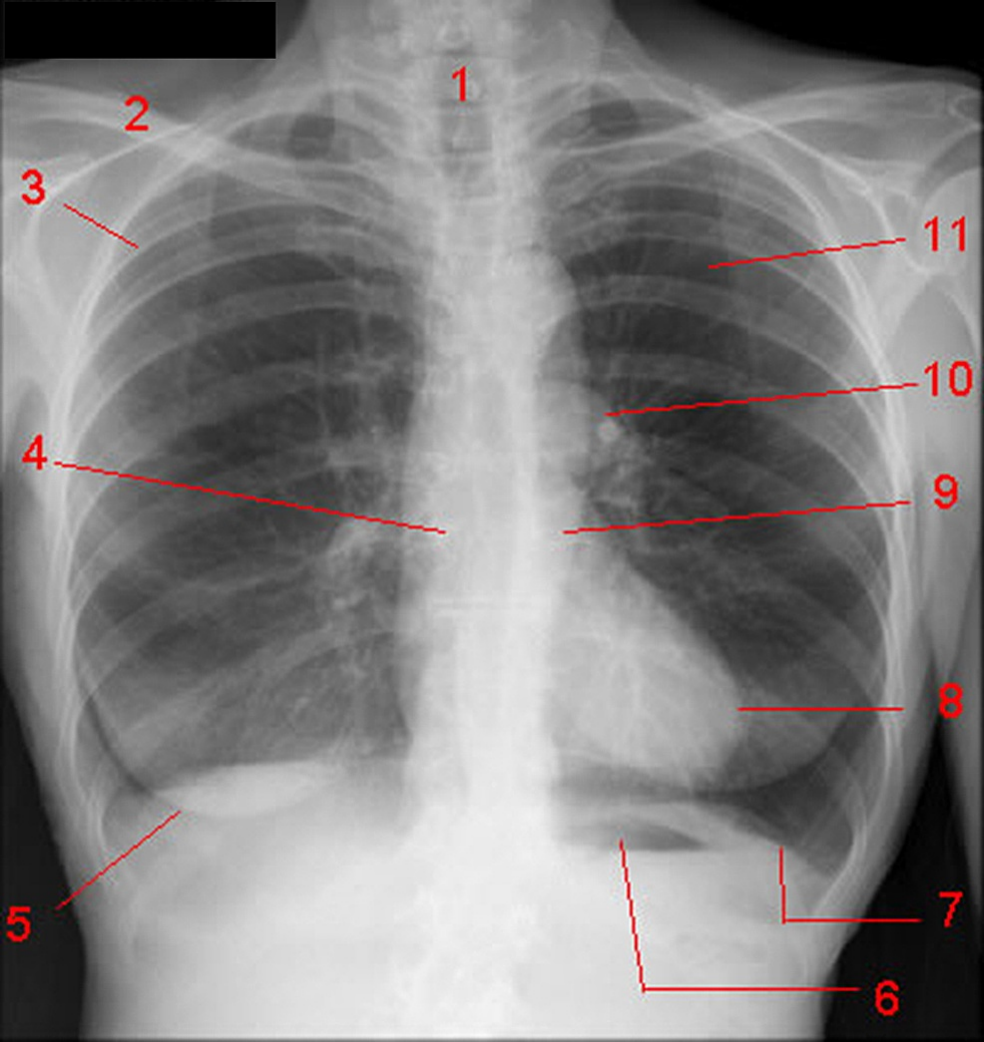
The image shows some anatomical areas that can be deduced from an X-ray of a healthy subject. 1. Trachea. 2. Clavicle. 3. Posterior portion of the fourth rib. 4. Right main bronchus. 5. Lower margin of the right breast. 6. Gastric air bubble. 7. Left diaphragmatic dome. 8. Left ventricle. 9. Descending aorta. 10. Left pulmonary artery. 11. Left lung.
